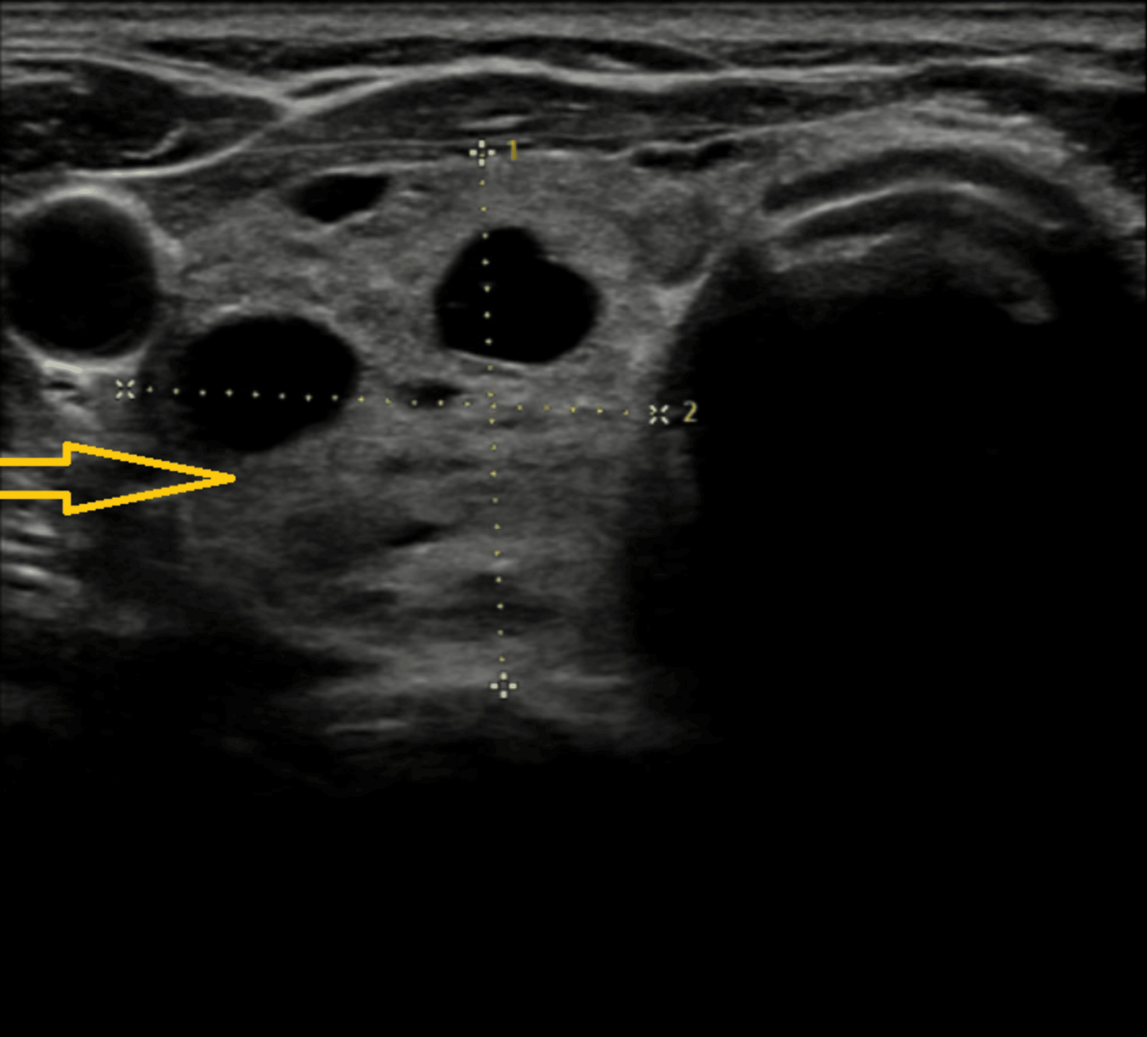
Transverse neck ultrasound view section revealing multiple U2 nodules in the right thyroid lobe
